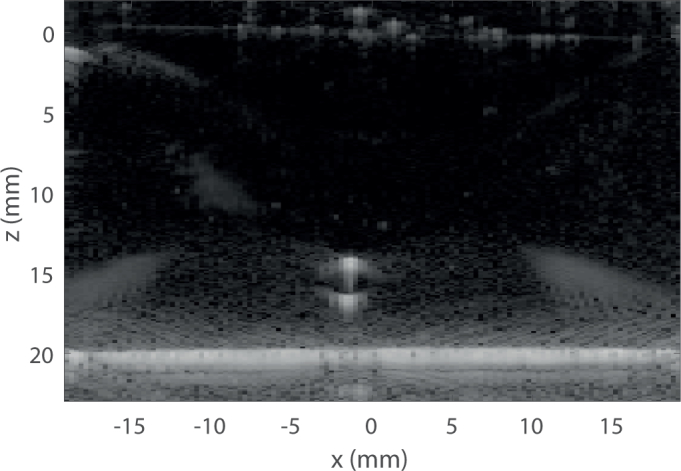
Ultrasound image of the experimental gel.
